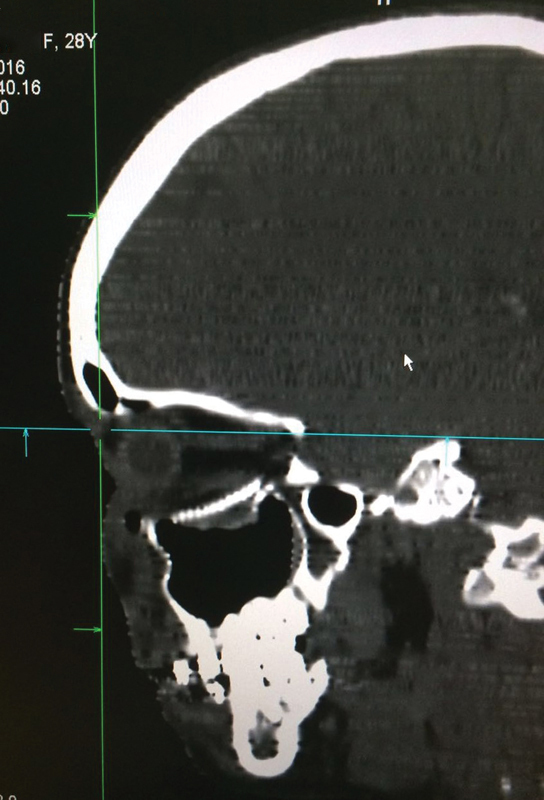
Computed tomographic (CT) scan 4 months postoperatively showing adequate
